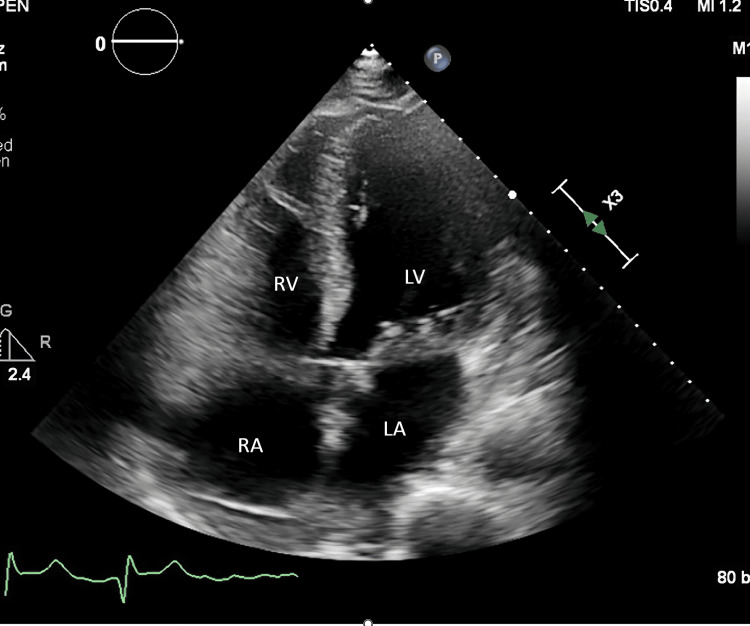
Echocardiogram of the patient showing akinetic apex and global hypokinesis of the left ventricle with regional variations RA, right atrium; LA, left atrium; LV, left ventricle; RV, right ventricle
